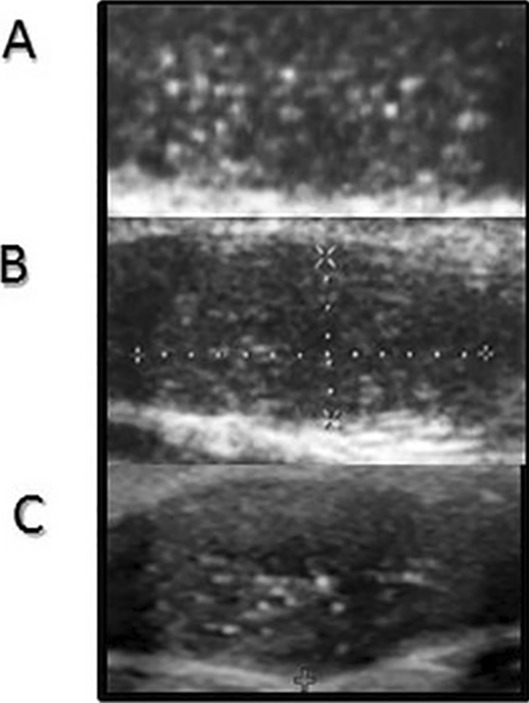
Ultrasound features of the testes during a follow-up of a prepubertal patient. A 2017 year (age 2.1 years), B 2019 year (age 4.1 years), C 2022 year (age 7.1 years)
